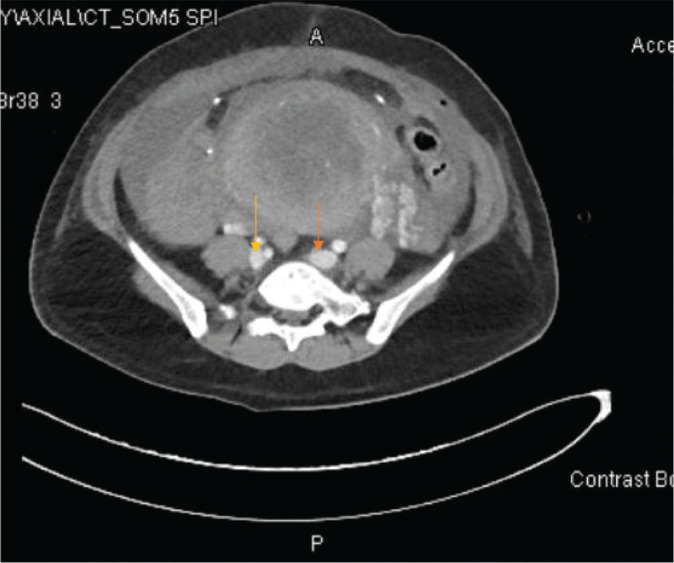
CT abdomen showing bilateral ovarian vein thrombosis.
